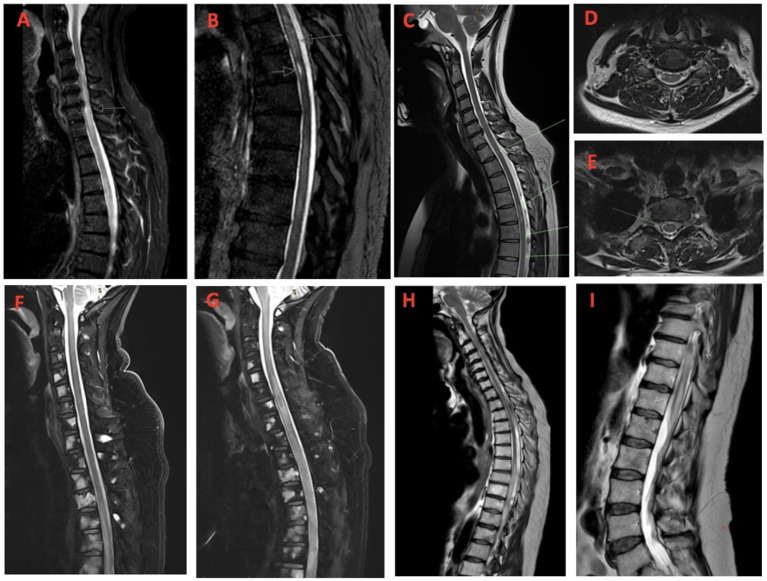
(A, B) Case 1-Sagittal MRI demonstrating eccentric patchy abnormal intramedullary high STIR signal and enhancement within the spinal cord, most conspicuous at the level of C6/C7 and T5 (arrows); (C) Case 2-Sagittal and (D, E) axial MRI images showing longitudinally extensive abnormal T2 signal hyperintensity in the cord from T4/T5 to T12 with a faint focus of cord signal hyperintensity at C5/C6 and at T1/2; (F) Case 3-Sagittal MRI images demonstrating longitudinally extensive abnormal T2 signal hyperintensity in the mid-thoracic cord on initial presentation and (G) extending to involve the mid- to lower cervical spinal cord 2 weeks later; (H) Case 4-Sagittal MRI images demonstrating longitudinally extensive abnormal T2 signal hyperintensity from mid-thoracic cord T6/T7, extending to the conus medullaris (I).
